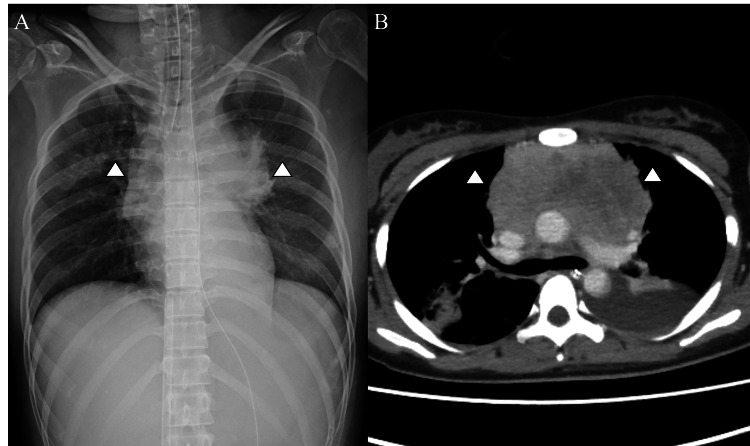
Imaging results on hospital day 9 A. Portable chest radiograph on hospital day 9. B. Contrast-enhanced CT on hospital day 9 The anterior mediastinal mass was reduced in size, resulting in the resolution of compression on the trachea, main bronchi, and pulmonary arteries. Consolidation was noted in the right upper lobe, likely representing airway-related inflammation such as pneumonia CT: computed tomography
